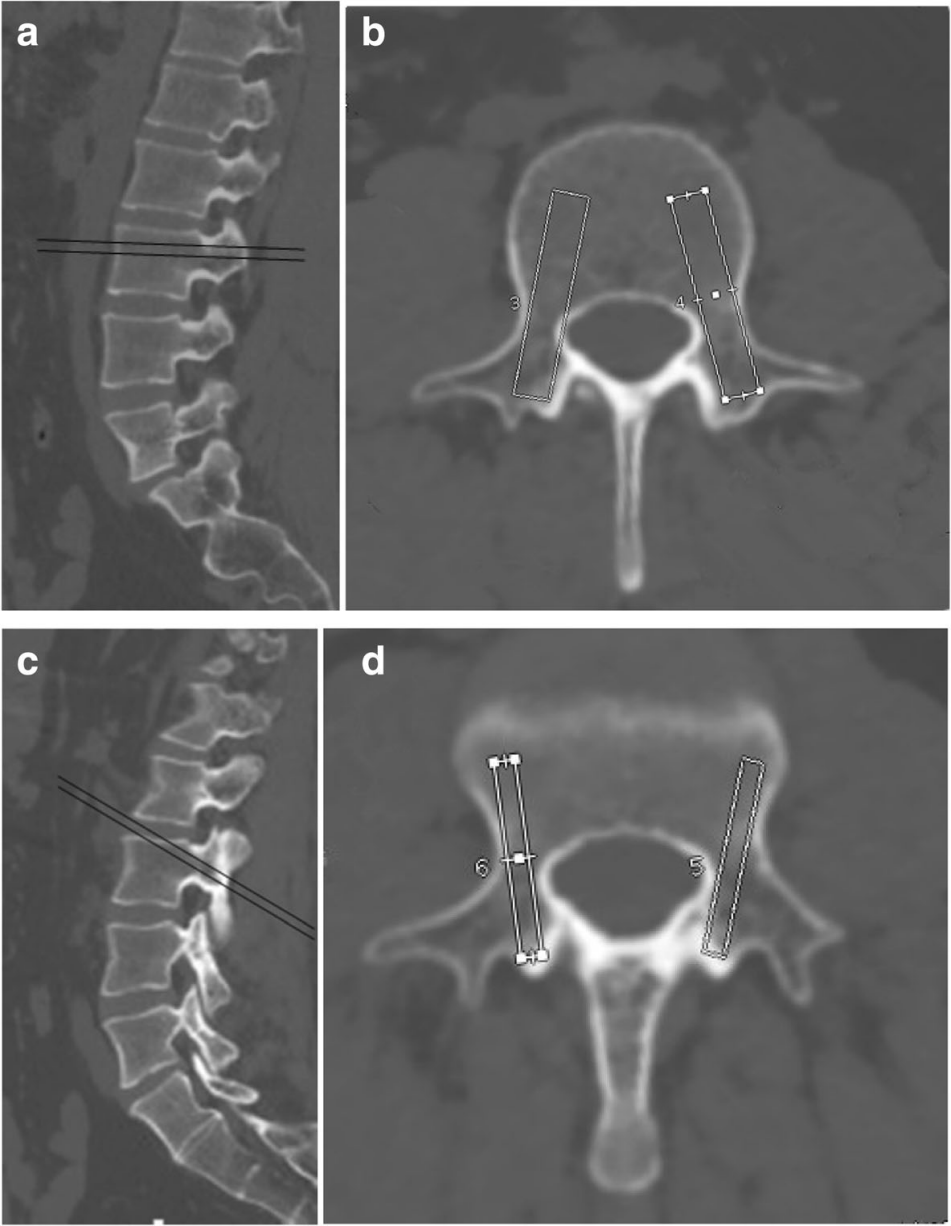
Sagittal thin-sliced CT images displaying the screw insertion angle for the TT (a) and CBT (c). ROI used for calculating the CT numbers for the TT (b) and CBT (d)
